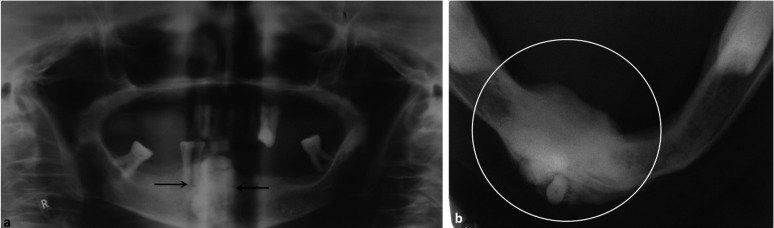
Orthopantomogram (a) showing radiopaque lesion in anterior mandible; mandibular occlusal view (b) showing the size and extent of lesion.
